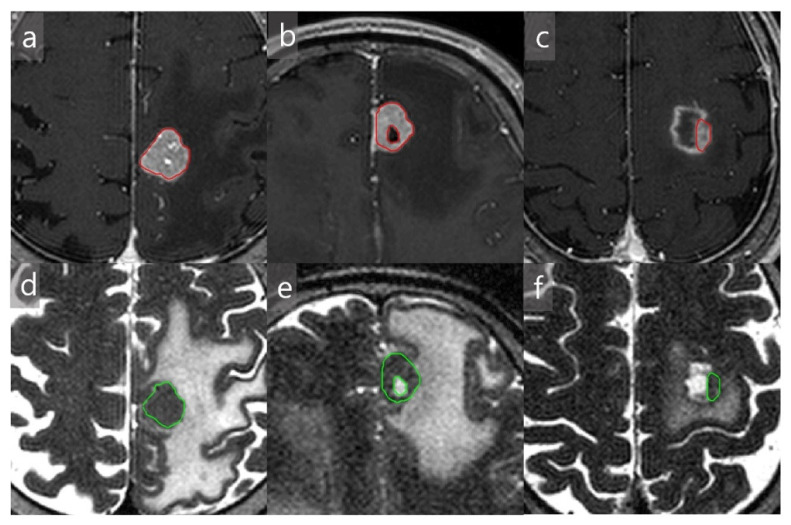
Examples of tumor segmentation on solid (a,d), predominantly solid (b,e), and predominantly cystic (c,f) metastases. The regions-of-interests are drawn on contrast-enhanced T1-weighted (a–c) and T2-weighted (d–f) images for each lesion.
